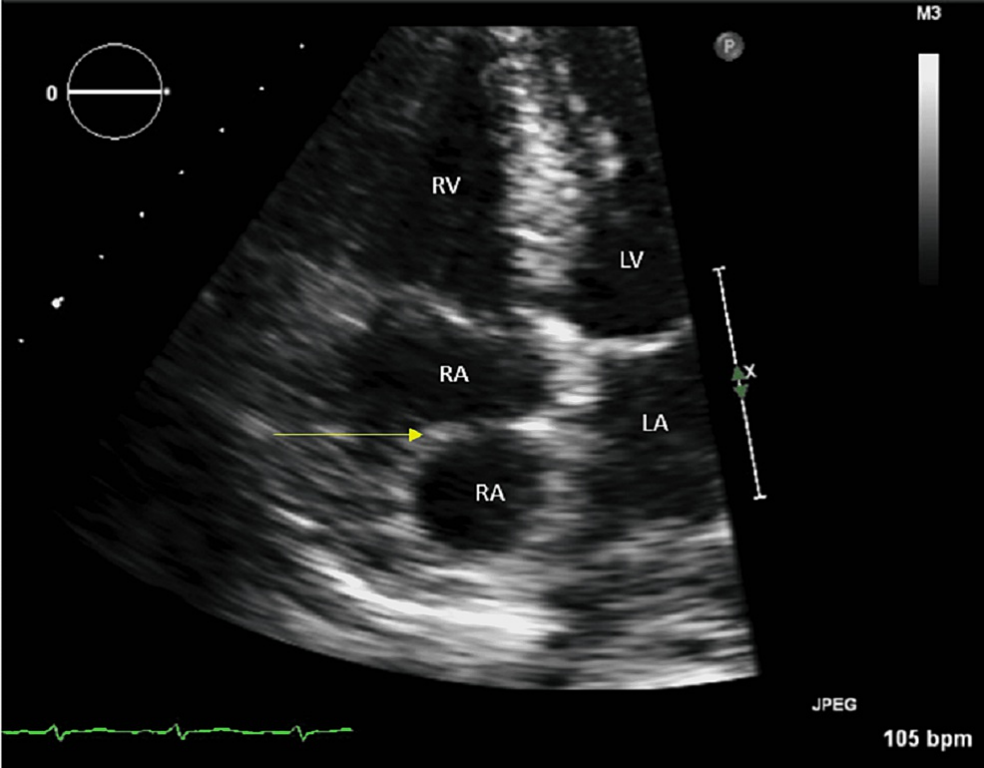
Transthoracic echocardiogram (TTE) ECHO showing a mass in the right atrium (yellow arrow) LA, left atrium; LV, left ventricle; RA, right atrium; RV, right ventricle
